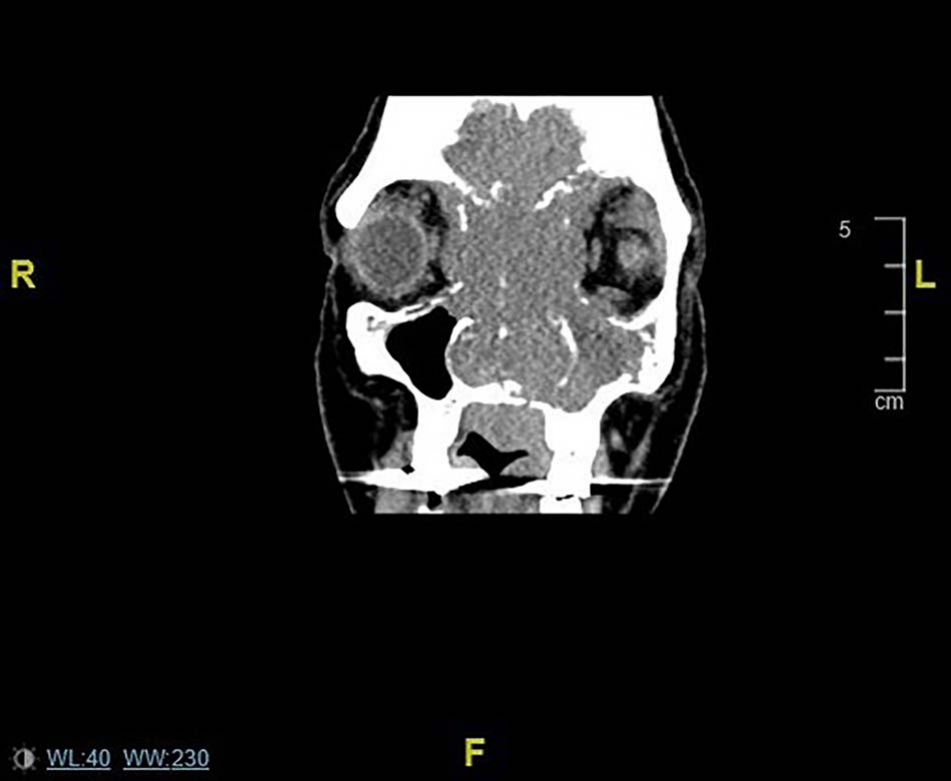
Coronal CT sinuses/head images displaying sinonasal mass invading anterior skull base, orbits and intracranially.
